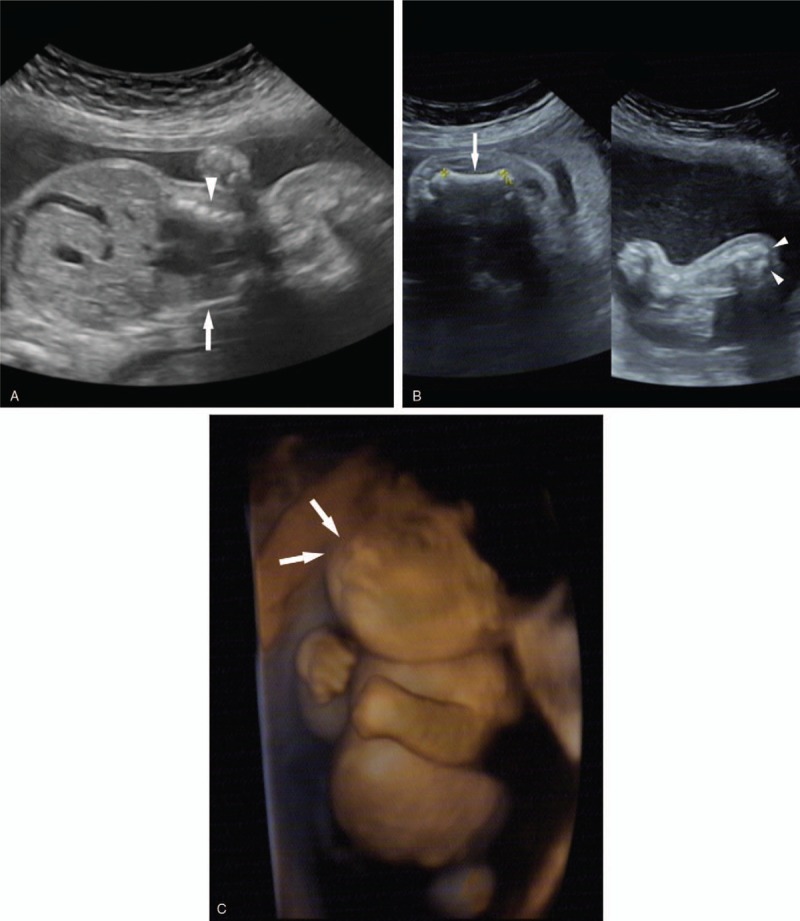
Ultrasound findings: (A) at gestational week 24+5: small and narrow thorax (arrowhead), chest circumference 15.6 cm, abdominal circumference 21.1 cm, shorter spine (arrow); (B) at gestational week 24+5: bending and short femur (arrows), thick metaphysis with high echoes (arrowheads); and (C) flat face and nose bridge (arrows).
